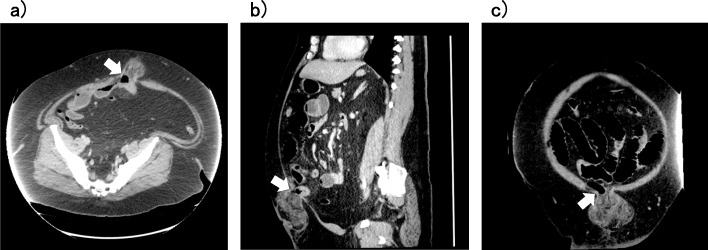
Abdominal contrast-enhanced CT. The greater omentum and small intestine appear incarcerated (arrow). a Horizontal section. b Coronal section. c Sagittal section
